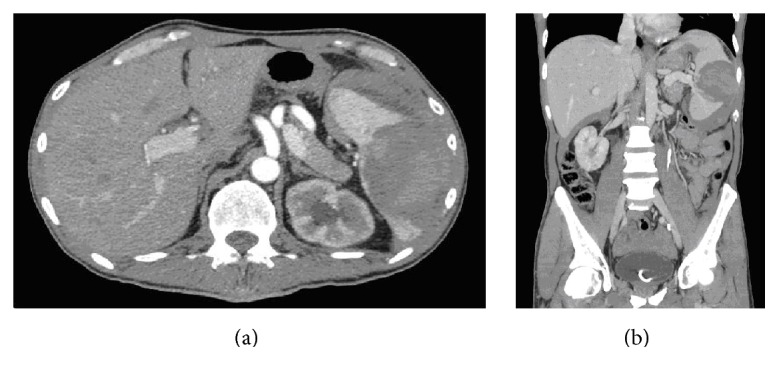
Axial CT scan demonstrating splenic bleeding (a); coronal scan (b).
